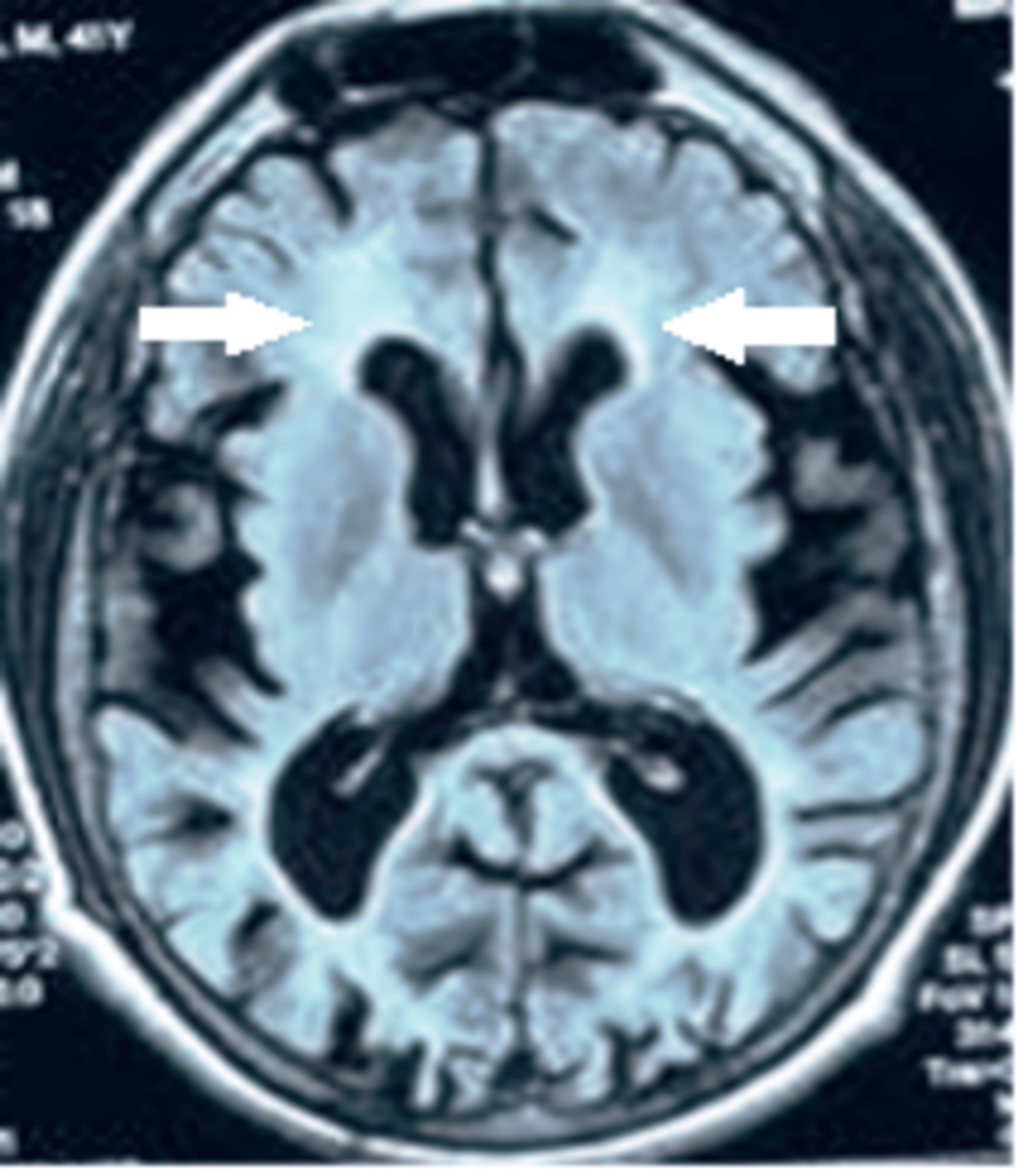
Periventricular T2/FLAIR hyperintensities with diffuse cortical atrophy
